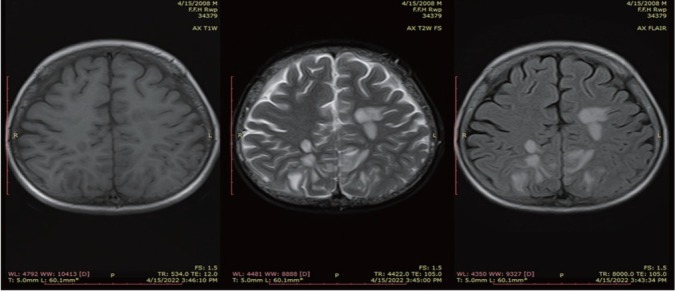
Axial T1WI, T2WI and FLAIR sequence showing areas of T2 and FLAIR hyperintensities in bilateral parietal lobes. T1WI, T1 weighted images; T2WI, T2 weighted images; FLAIR, fluid attenuated inversion recovery.
